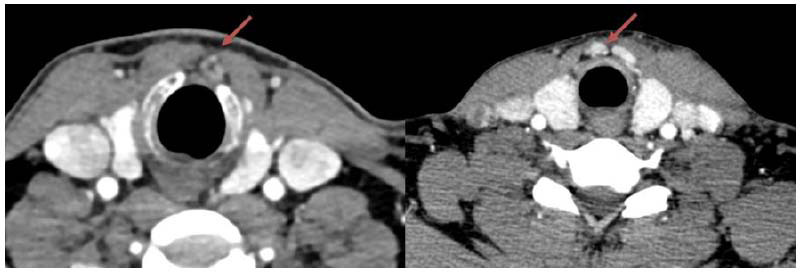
CT scan showing DLN in two patients, indicated by the red arrows.
